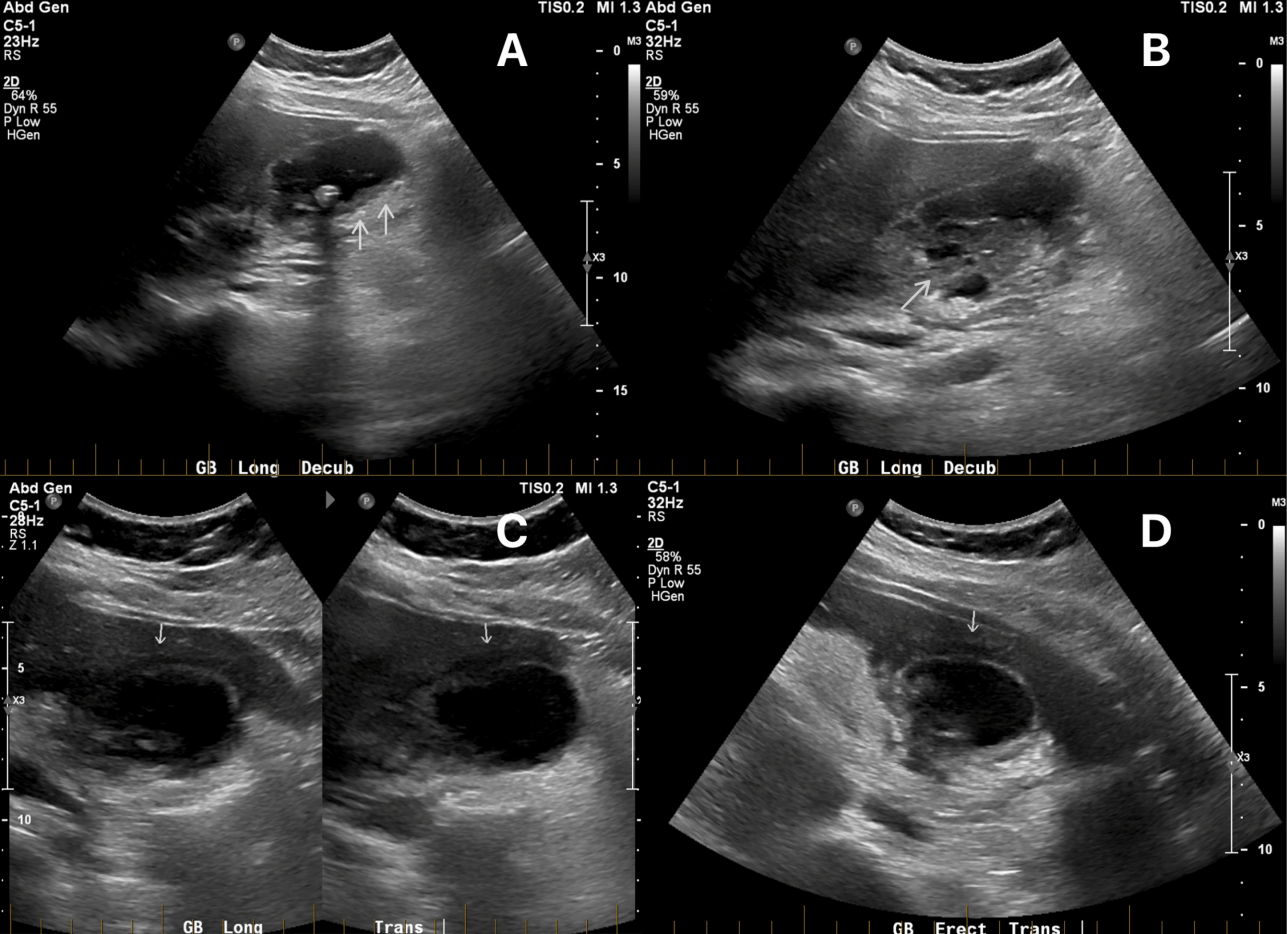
Ultrasound of the gallbladder on the second presentation. While initially reported as typical acute calculous cholecystitis with a thickened gallbladder wall of 3.78 mm, features of XGC are noted in retrospect. The multiple hypodense nodules occupying a large area of the thickened gallbladder wall are suggestive of XGC (white arrows). Also seen is a calculus within the gallbladder body (A).
